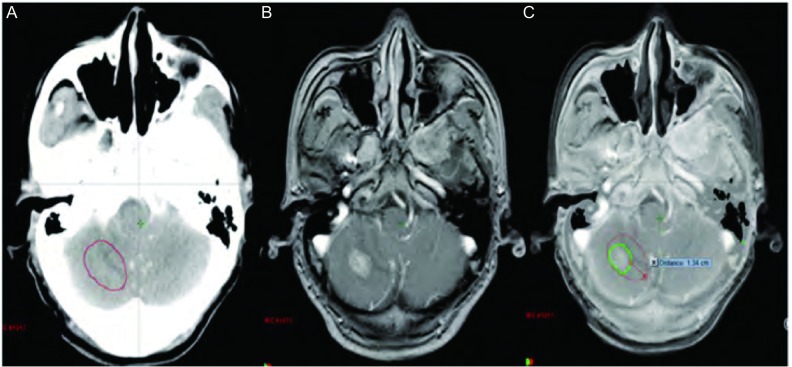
幕下转移灶（A：增强CT图像；B：MRI图像；C：CT/MRI融合图像）。 Infratentorial metastasis (A: Enhanced CT image; B: MRI image; C: CT/MRI fusion image).
